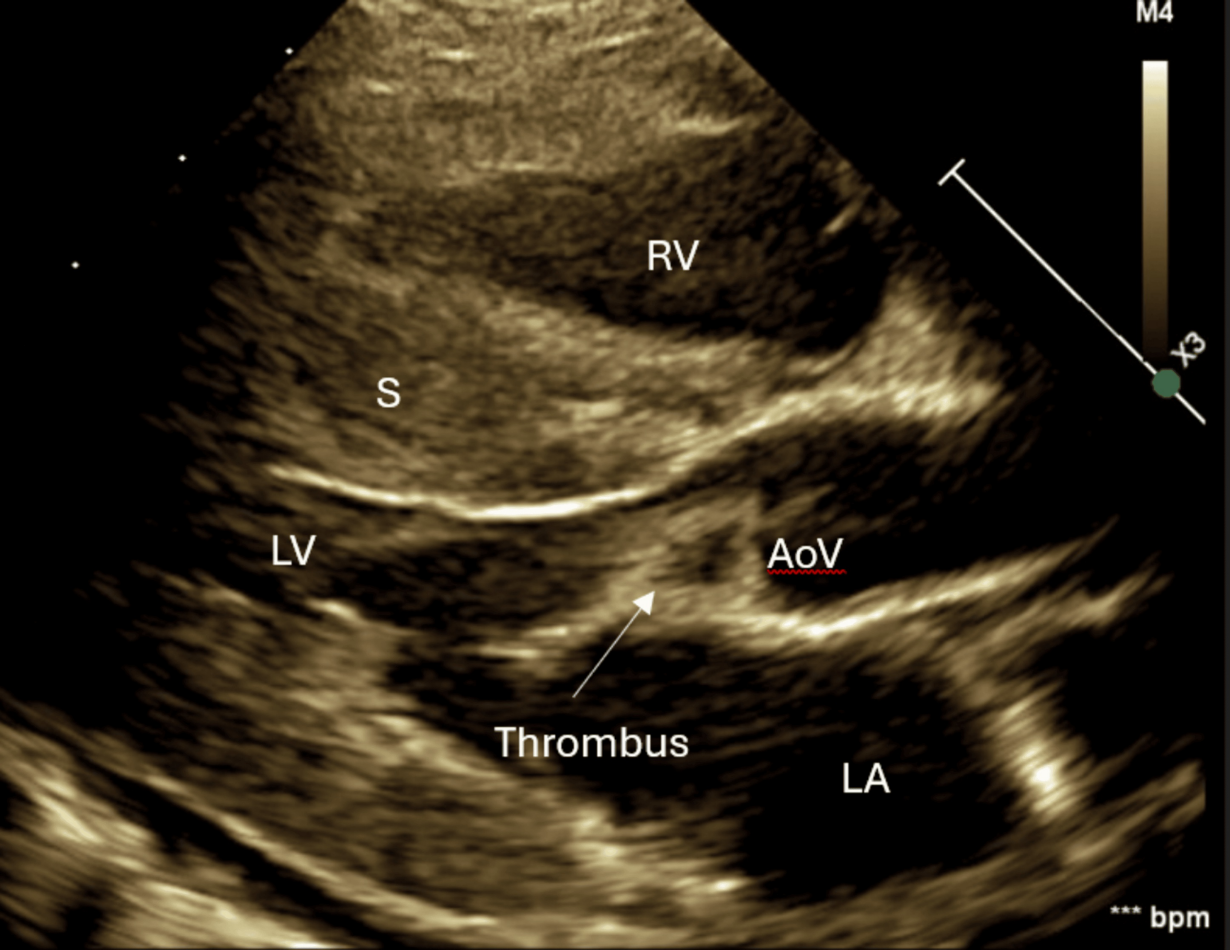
Echocardiogram showing the presence of thrombus RV: right ventricle, S: septum, LV: left ventricle, LA: left atrium, AoV: aortic valve
